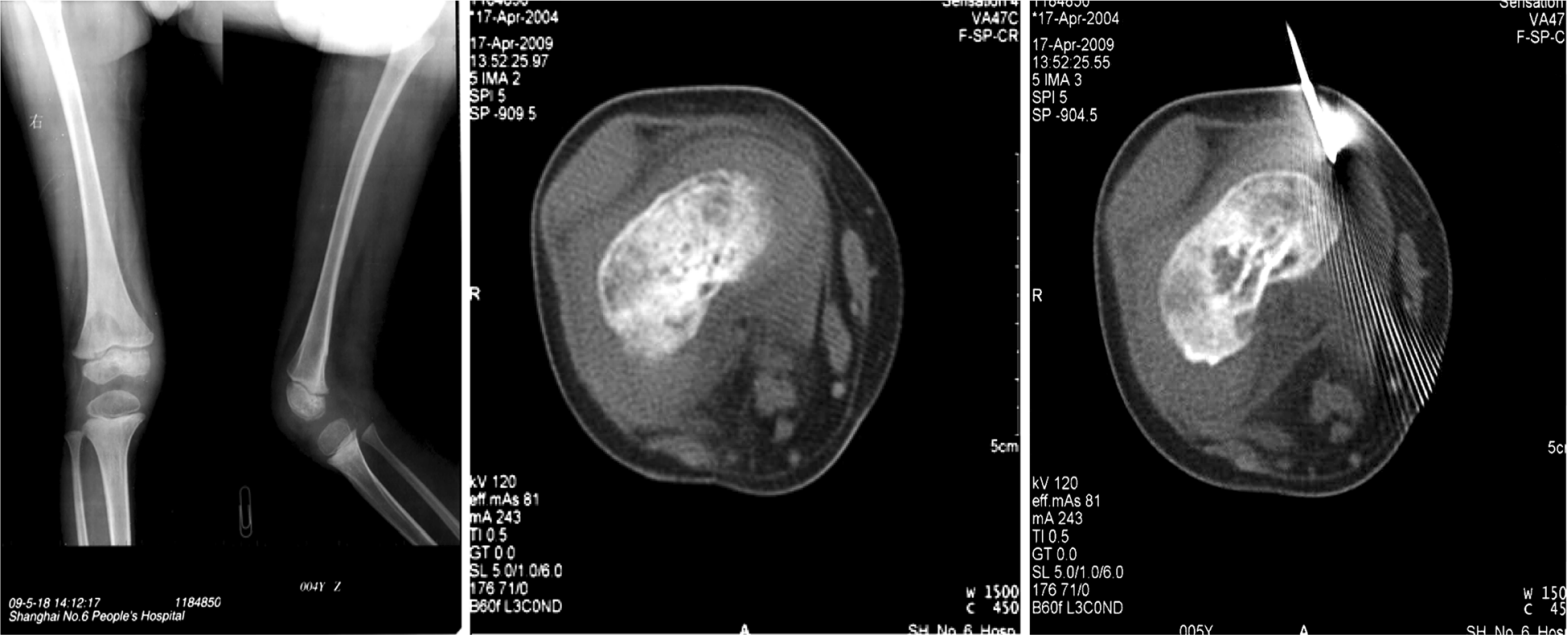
X-ray and CT imaging of the right leg of the case. A. X-ray showed that the pattern of bone was generally normal and there was no swelling of surrounding soft tissue. Density of the metaphysis of the lower right thighbone had irregularly reduced. B and C. CT scan showed the density of medullar of the lower right femoral metaphysis was asymmetrically reduced with irregular thickening of the cortex and swelling of soft tissue.
